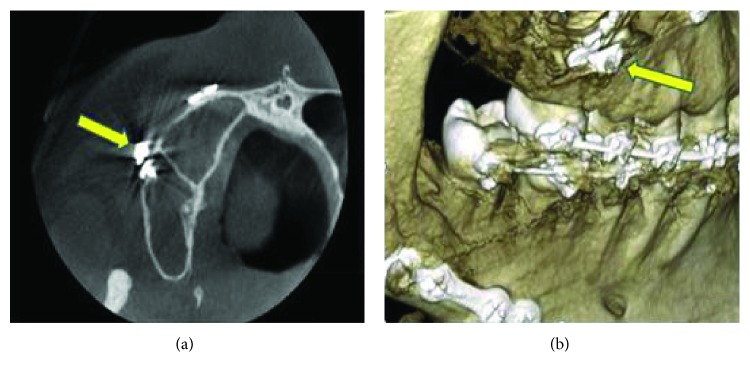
Cone Beam CT images showing the dislodged molar tube lying outside the right maxillary antrum, as indicated by the arrow in axial view (a), while its position in relation to the rigid plate and screws on the zygomatic buttress can be seen clearly in 3-D image (b).
